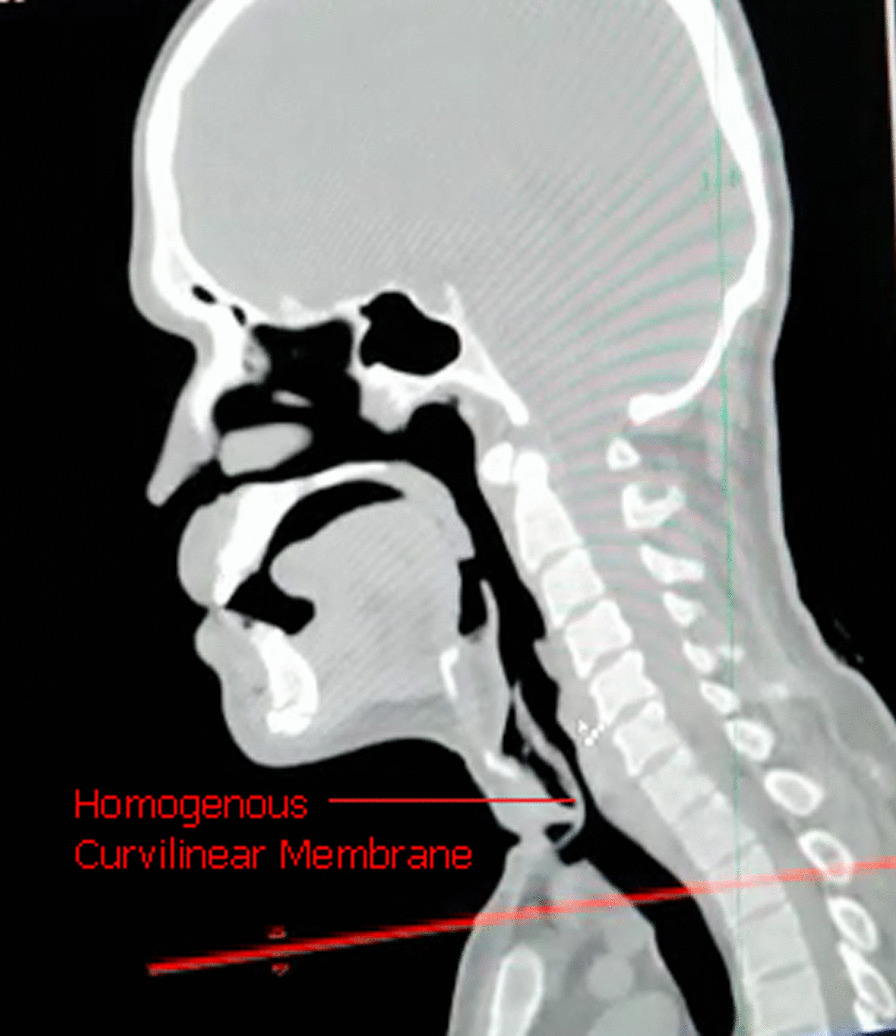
Homogenous curvilinear soft tissue density in the hypopharynx, larynx, and upper trachea causing mild to moderate luminal narrowing seen on CT scan of the neck
